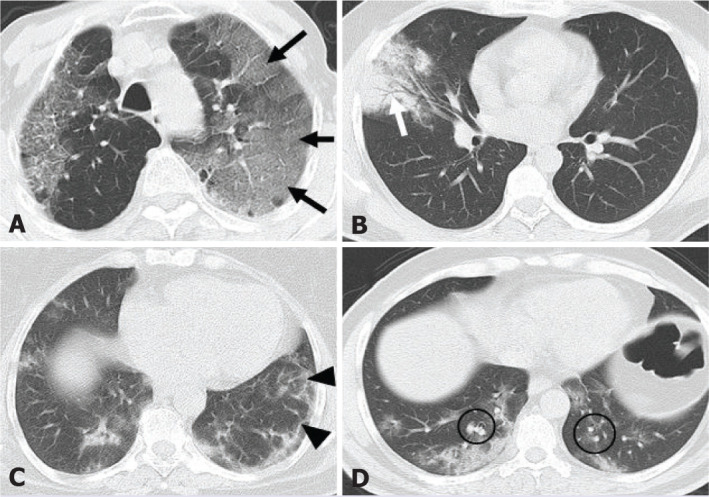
The axial section of the chest computed tomography scan shows typical findings (A) ground glass opacity, crazy paving pattern (black arrows); (B) consolidation, air bronchogram (white arrow); (C) reticular pattern (black arrow-heads); (D) pulmonary vascular enlargement (black circle).
